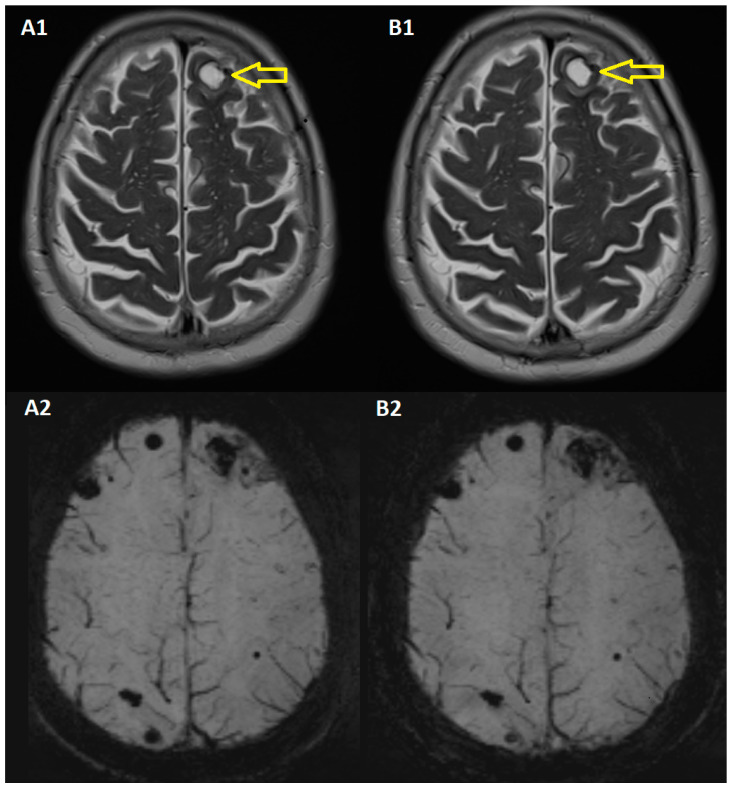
Brain Magnetic Resonance Imaging in October 2021 T2 (A1), SWI (A2) vs. December 2021 T2 (B1), SWI (B2): In the MRI from October 2021 (images (A1,A2)), the frontal lesion is noticeable and multiple lesions are observed, primarily on the brain’s convexity, including both gyral and cortical-subcortical junction lesions. In the MRI from December 2021 (images (B1,B2)) were similar lesions. Yellow arrows: Subcortical lesion.
